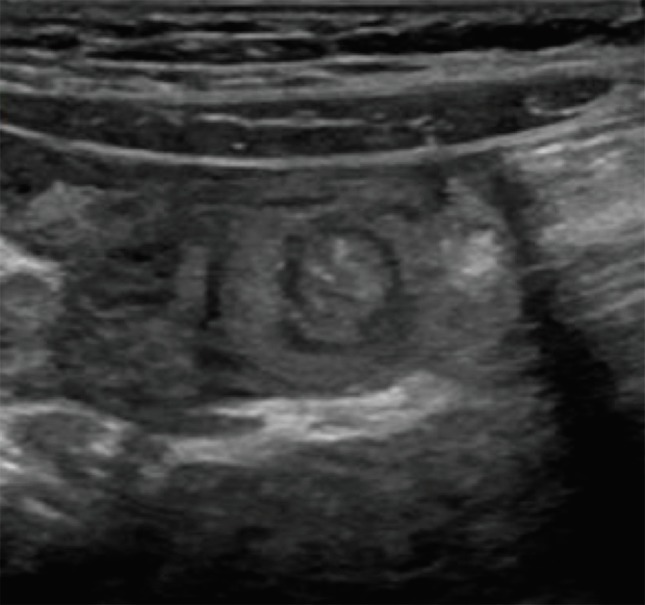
Abdominal ultrasound demonstrating the classic “target sign” of intestinal intussusception (white arrow)
